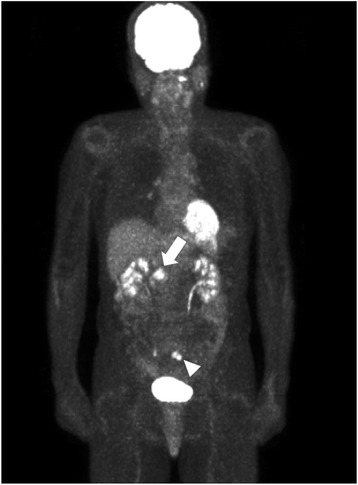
Preoperative findings of ^18^FDG-PET. The enlarged retroperitoneal lymph node has a maximum standardized uptake value (SUV max) of 6.08 (*arrow head*), and the pancreatic tumor has an SUV max of 7.83 (*arrow*)
